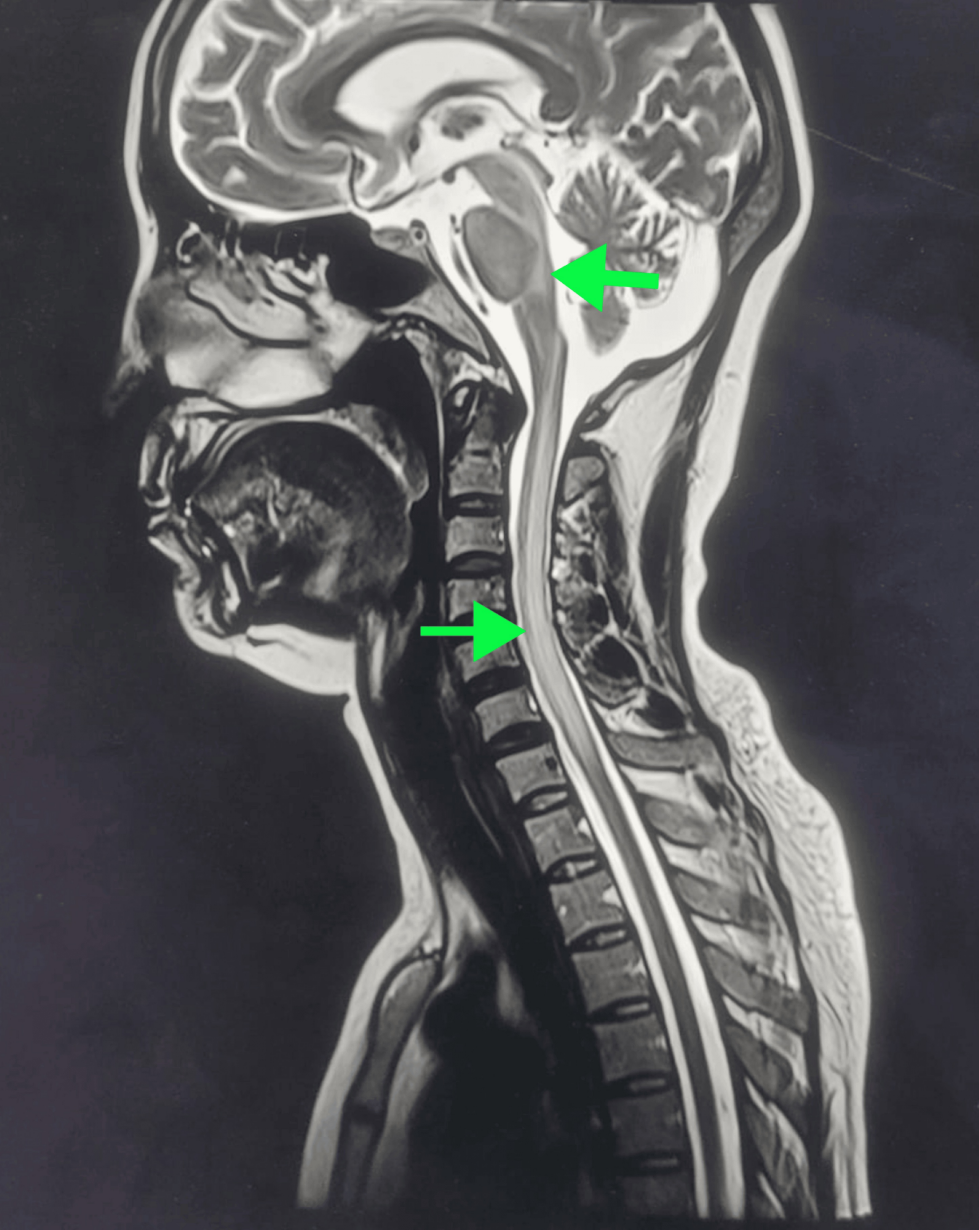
Sagittal T2-weighted MRI with gadolinium contrast showing high signal intensity in the brainstem and C1-C7 spinal cord segments.
